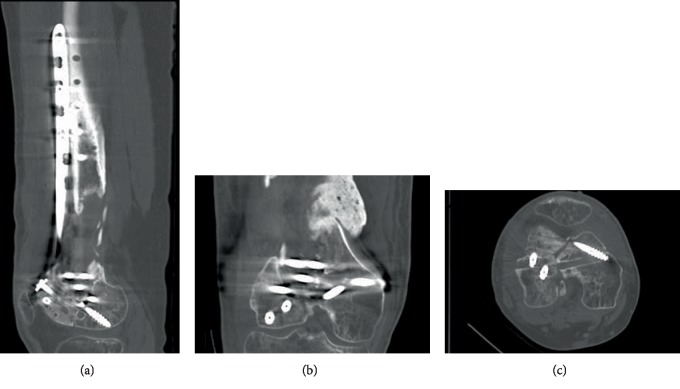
Postoperative CT scan of the right knee. Sagittal (a), coronal (b), and axial (c) CT scan of the knee shows the correction of articular step-off.
